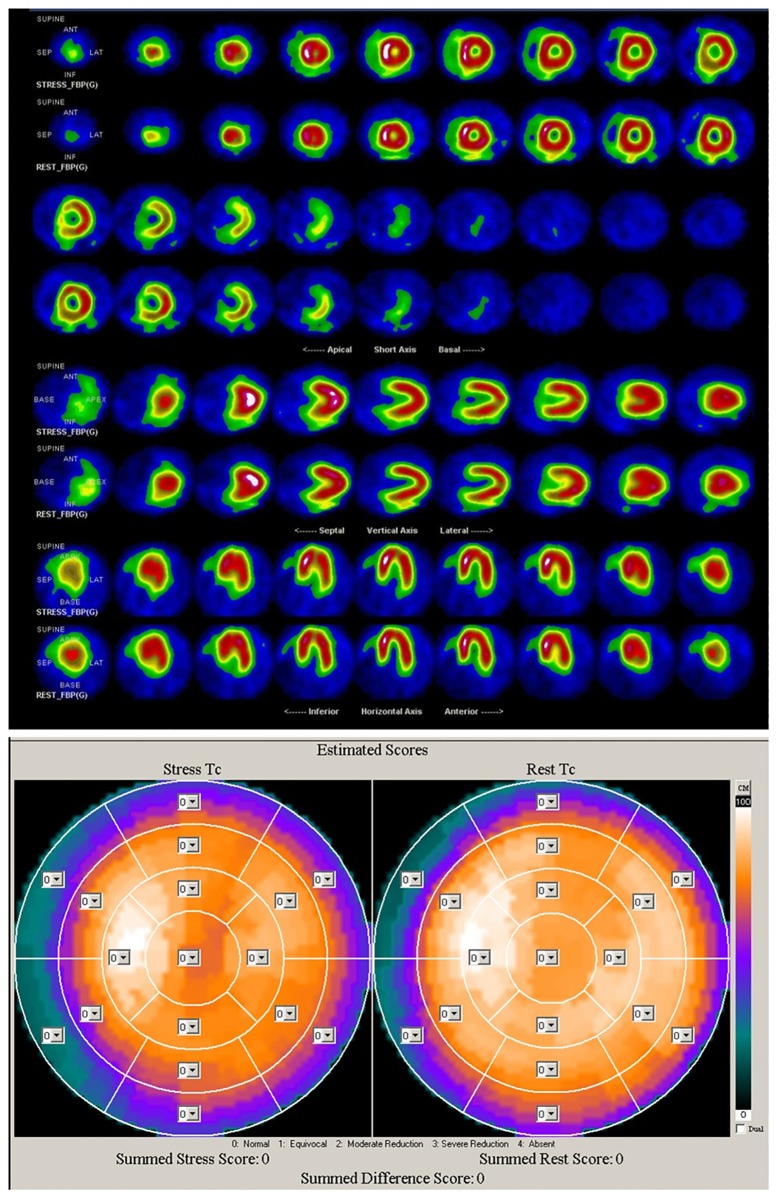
SPECT MPI results of GB0515. Both rest and stress SPECT ischemia score of GB0527 are 0.
